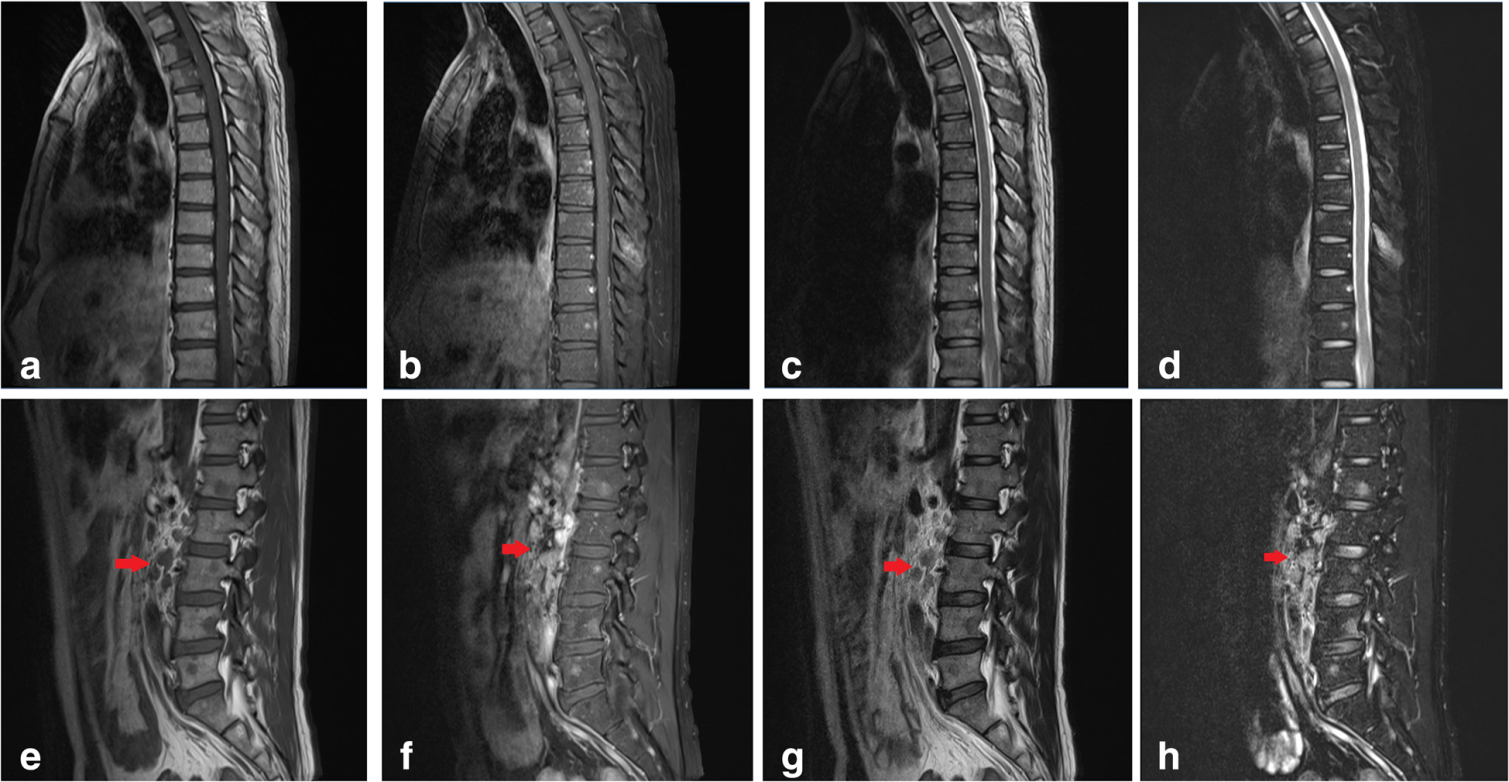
The magnetic resonance imaging (MRI) of the thoracic and lumbar vertebra was performed. Evidence of diffuse osseous metastatic disease in the thoracic and lumbar vertebra and multiply retroperitoneal lymph node metastases which were mixed together as masses (arrow). a-d: imaging of thoracic spine; a: T1 weighted imaging (T1WI); B: T1WI + FS (fat suppressed sequence); c: T2 weighted imaging (T2WI); d: T2WI + FS. e-h: imaging of lumbar spine; e: T1WI; f: T1WI + FS; g: T2WI; h: T2WI + FS
